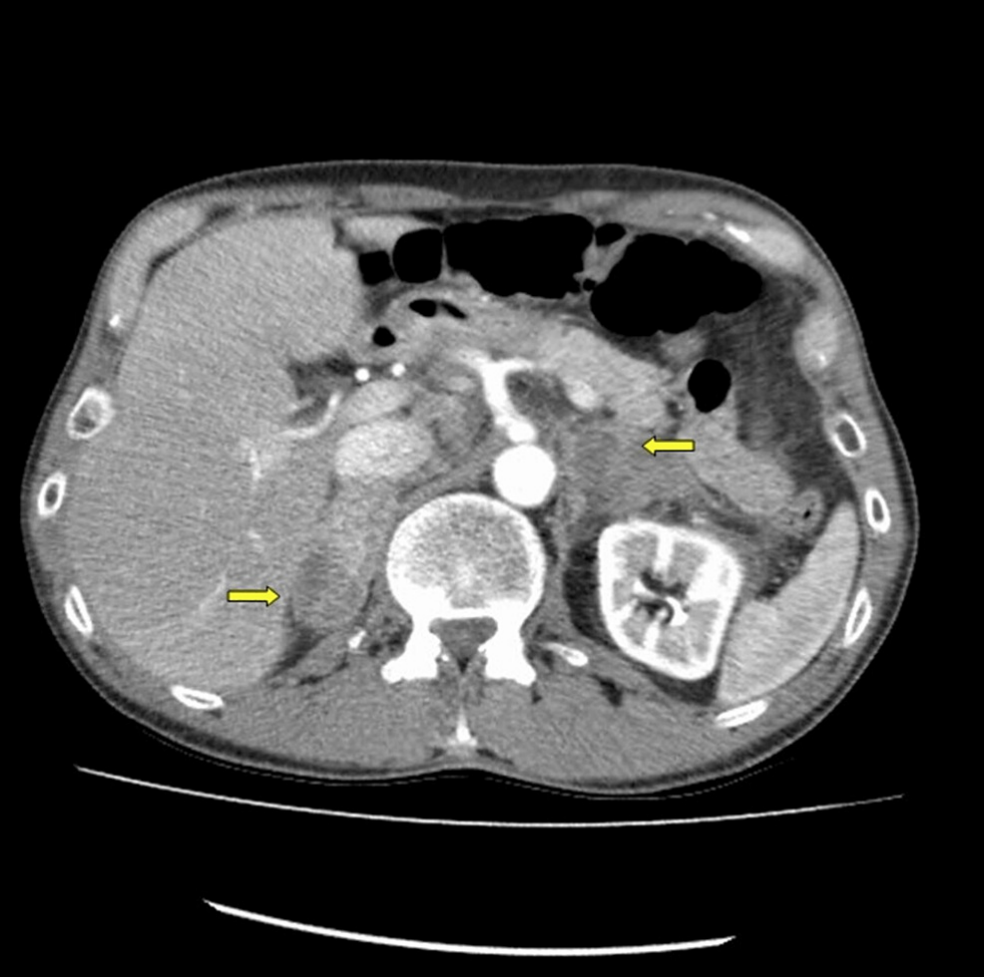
CT abdomen with contrast: bilaterally enlarged adrenals with hemorrhage and periadrenal infiltration (yellow arrows)
